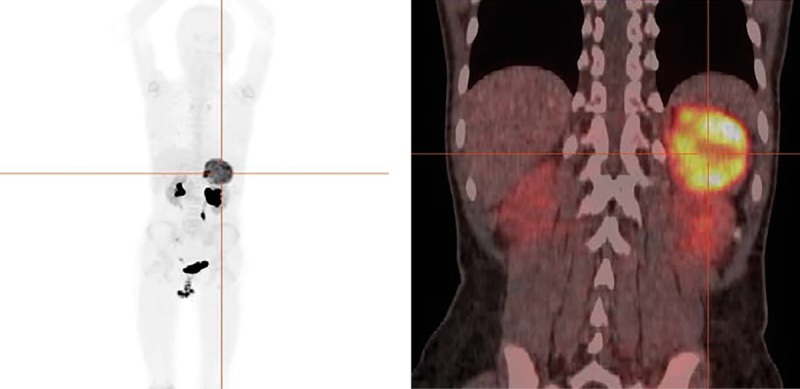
Рисунок 4. ПЭТ/КТ всего тела с 18F-ДОФА. В левом надпочечнике визуализируется ДОФА-позитивное образование размерами 105х67 мм и SUVmax=8,85, с выраженной неоднородной структурой за счет многочисленных разнокалиберных участков жидкостной плотности. Данных за наличие других патологических очагов нет.
